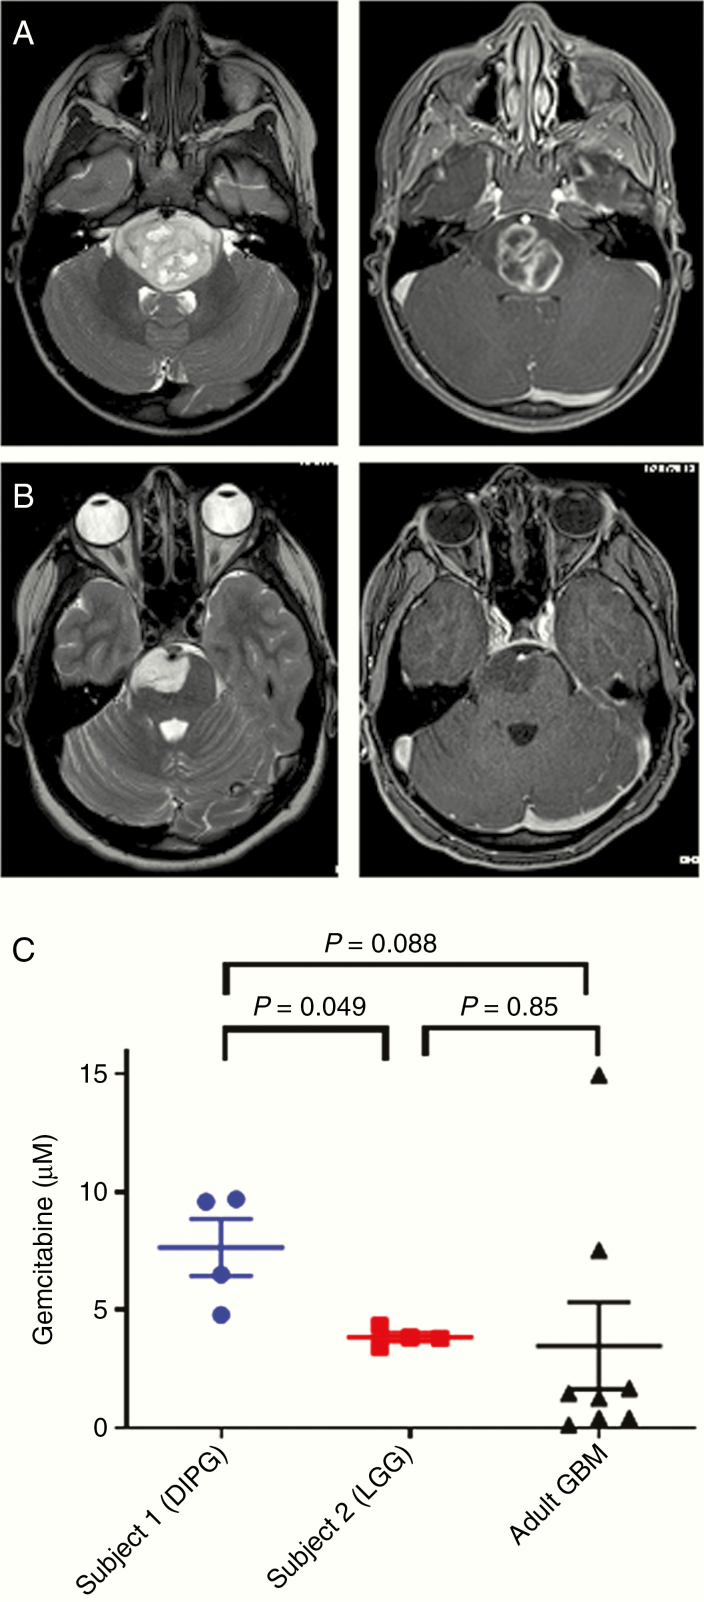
Clinical trial results. (A and B) T2 (left) and T1 post-contrast (right) axial magnetic resonance images of pontine tumors in patients 1 (A) and 2 (B). (C) Intratumoral gemcitabine concentrations in phase 0 clinical trial subjects compared to published values in adult glioblastoma patients. Error bars represent SEM.
